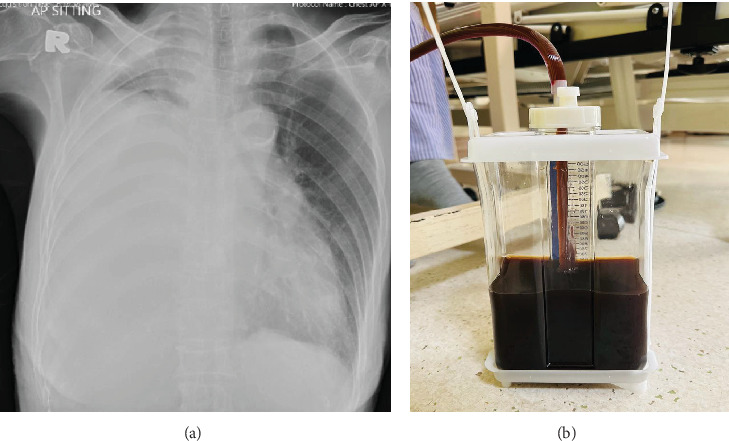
(a) Chest radiograph on arrival showed a right pleural effusion. (b) Dark brownish fluid was drained from the right pleural cavity.
